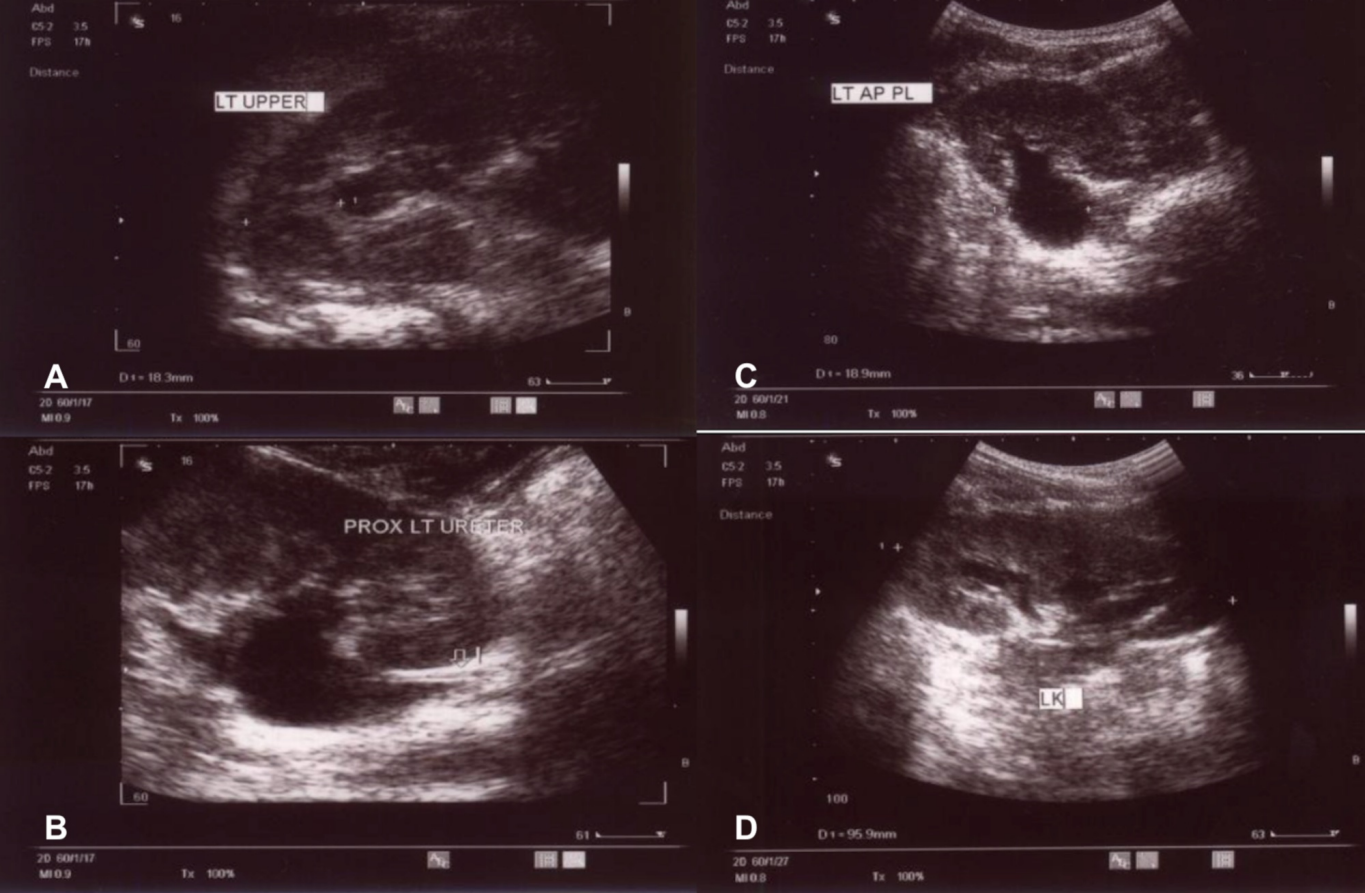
The Postoperative Ultrasound Scan A postoperative ultrasound scan showing normal findings. (A) Left upper ureter. (B) Left proximal ureter. (C) Left renal pelvis. (D) Left kidney.
